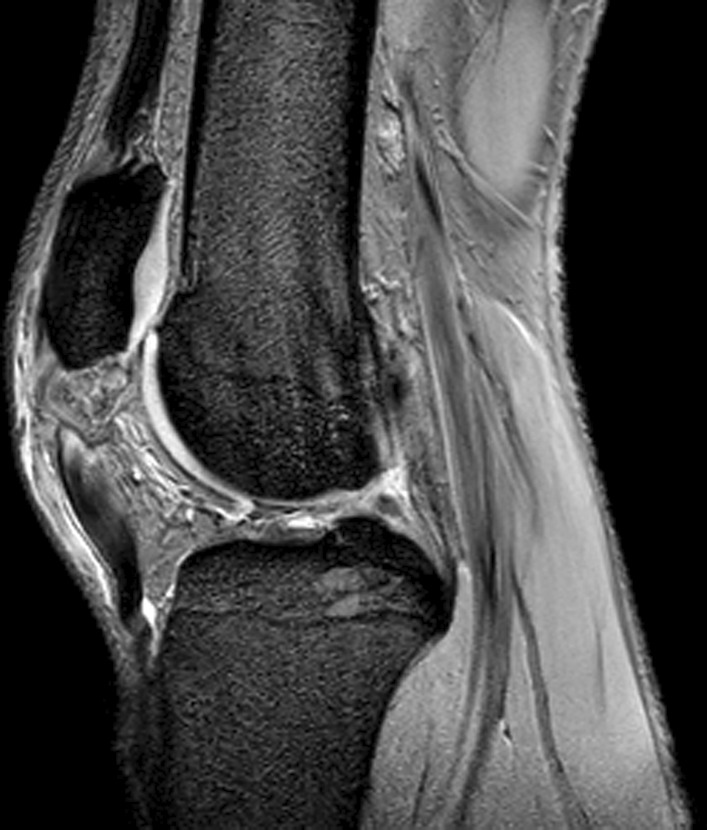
Sagittal gradient echo MRI image that shows patella-tendon complete proximal rupture. Proximal retraction of the patella
